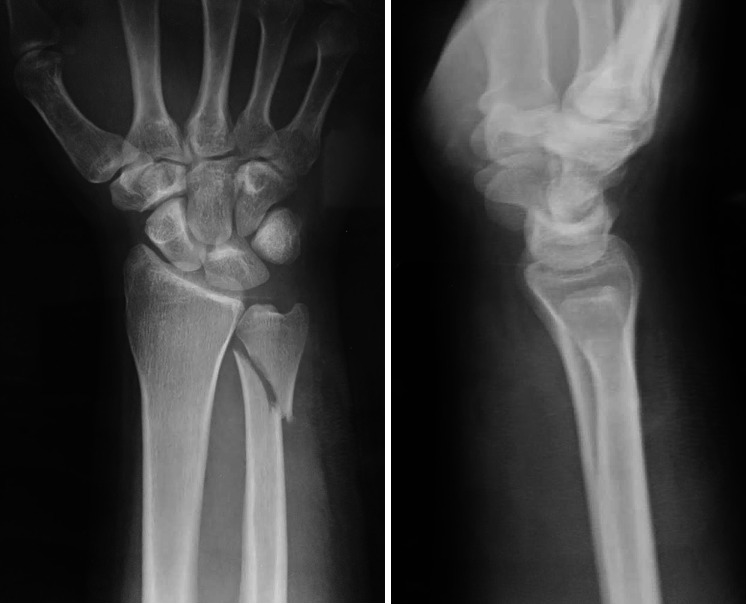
X-ray wrist anteroposterior and lateral view of a 48-year-old male patient with unstable distal ulnar fracture.
